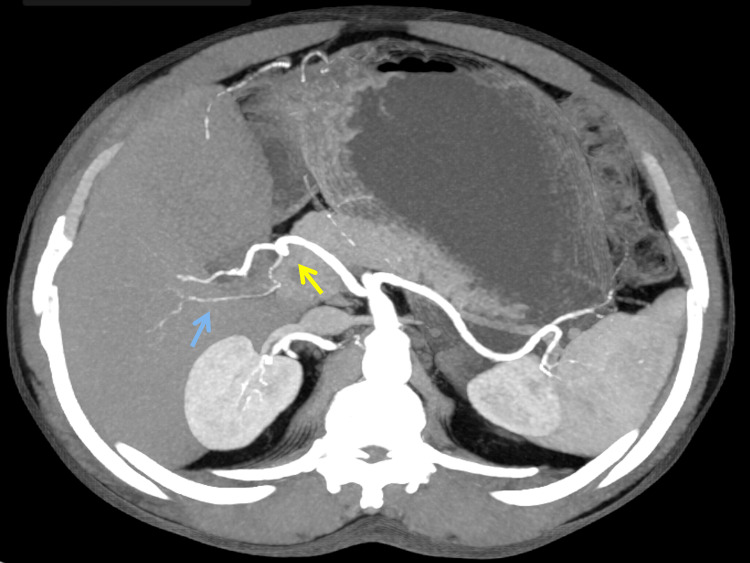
Contrast enhanced CT of the abdomen shows the replaced segment 6 artery (blue arrow) from the gastroduodenal artery (yellow arrow).
